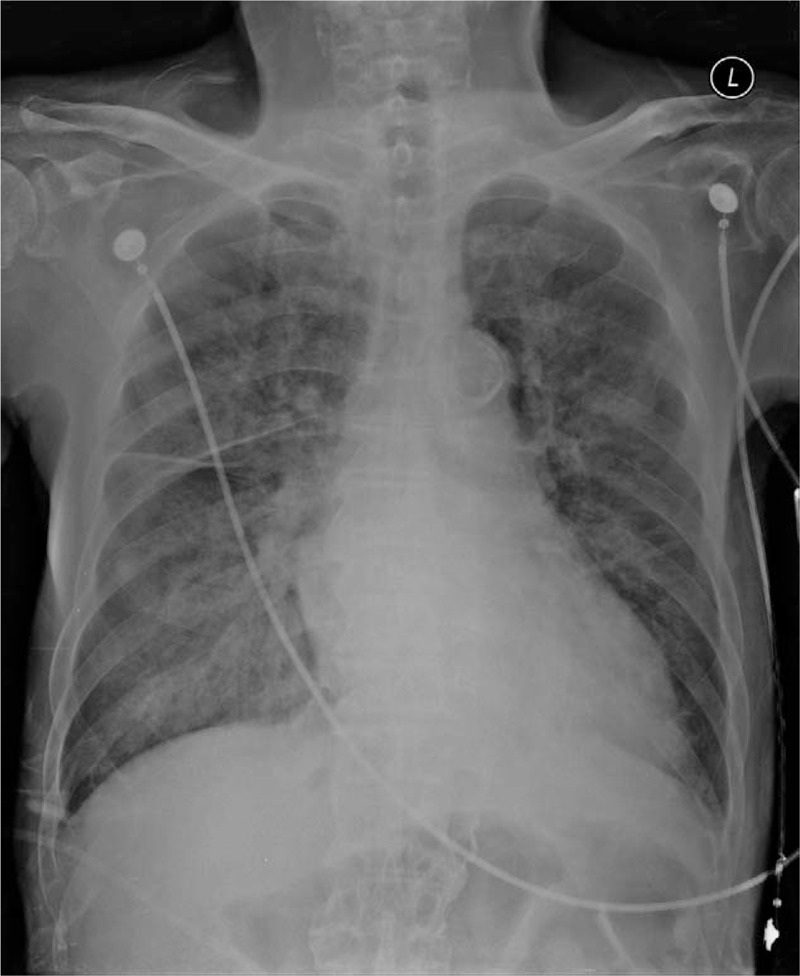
Postoperative chest x-ray showing bilateral vascular congestion.
